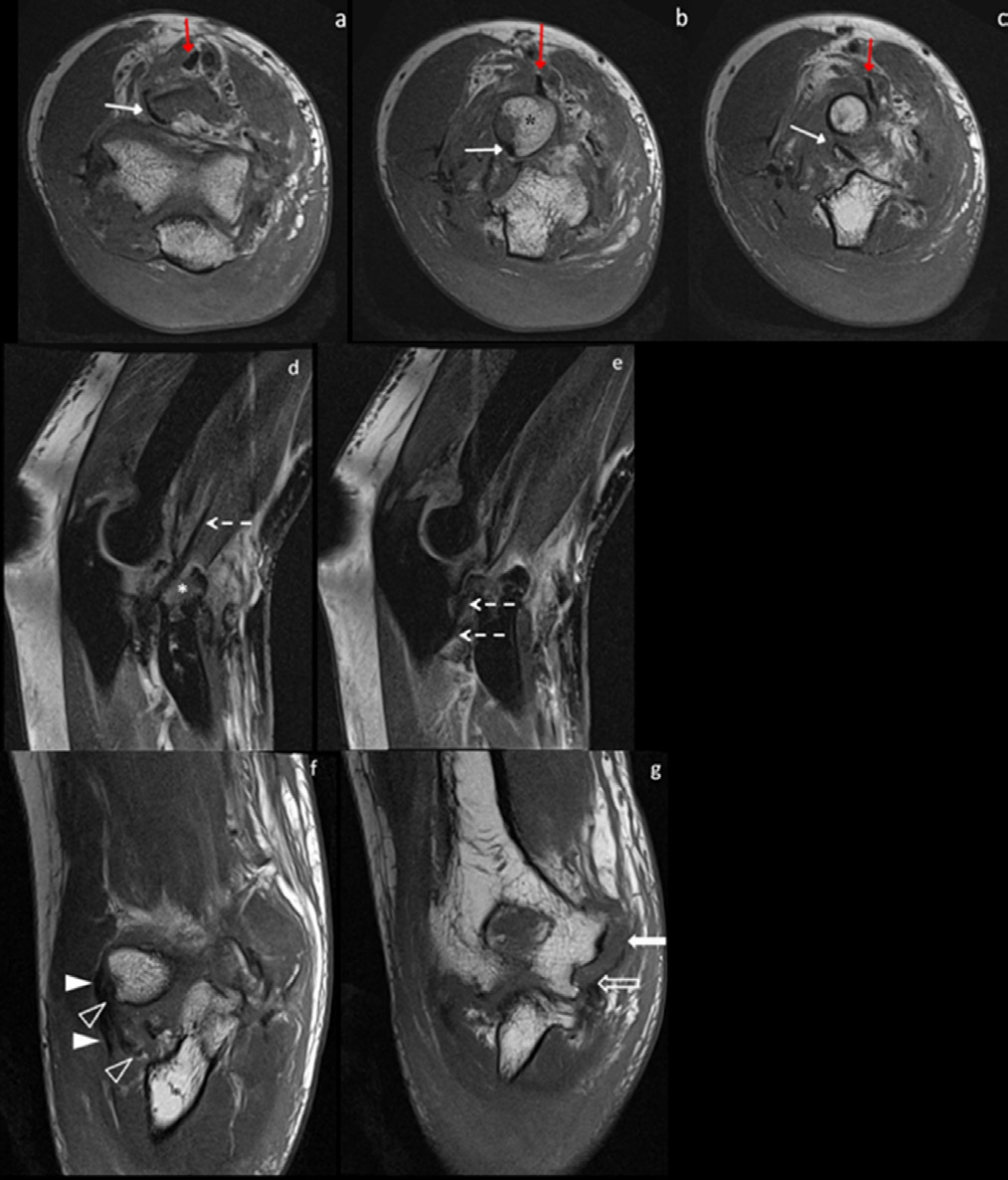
Multiplanar noncontrast MRI scan of the right elbow. (a–c) Axial contiguous *T*_1_ weighted images from proximal to distal across the level of the radial head demonstrate the brachialis tendon (*white arrow*) traversing posterolateral to the radial head (*) to insert into the coronoid process at the tuberosity of the ulna (c). Radial head fracture with associated fragments within the radial notch of the ulna are again seen. The biceps tendon (*red arrow*) is also identified anterior to the radial head. The annular ligament is not seen encircling the radial head, and is completely torn. (d–e) Sagittal Proton Density-weighted fat saturated images of the right elbow demonstrate the brachialis tendon (*Dashed arrows*) along the sagittal plane coursing posterior to the radial head fracture-dislocation (*). (f - g) Coronal *T*_1_ weighted images show disruption of the lateral collateral ligamentous complex in (f) and medial collateral ligaments in (g). (f) Proximal common extensor tendon (*solid arrowhead*) shows high grade partial tear at its origin at the lateral humeral epicondyle and is wavy distally, probably partially torn. The RCL (*open arrowhead*) shows high grade partial tear at its origin at the lateral humeral epicondyle and is completely avulsed from the annular ligament. These findings were confirmed intraoperatively. (g) The medial collateral ligament (*open block arrow*) and common flexor-pronator tendon (*solid block arrow*) show fibers discontinuity at the origin of the medial humeral epicondyle, reminiscent of complete tears. These findings were also confirmed intraoperatively. Extensive subcutaneous, intermuscular and intramuscular oedema are seen across all of the above images. RCL, radial collateral ligament.
